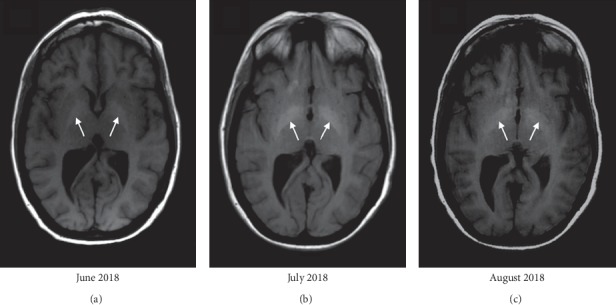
Axial T1-weighted MRI brain performed serially over three months, showing the progression of the T1 hyperintensities in the globus pallidi from June 2018 to July 2018, followed by the improvement of the T1 hyperintensities in August 2018, one month after discontinuation of TPN. (a) Evidence of developing T1 hyperintensities in the bilateral globus pallidi on a previous hospital admission in June 2018. (b) Further increase in signal in the bilateral globus pallidi on T1-weighted images in July 2018, corresponding to her pronounced Parkinsonian features on clinical presentation. (c) Improvement in the T1 hyperintensities in the globus pallidi, collected one month after cessation of TPN use and corresponding to near-resolution of her Parkinsonism.
